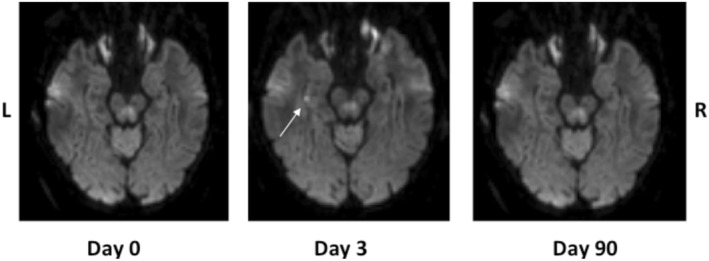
Evolution of a typical hippocampal lesion in one patient. Day 0: Initial brain MRI performed with high‐resolution diffusion‐weighted imaging during the acute phase is normal. Day 3: High‐resolution diffusion‐weighted imaging obtained 3 days after symptom onset shows a small, round, hyperintense lesion in the right hippocampus (arrow), which was no longer visible 90 days after onset on Day 90.
